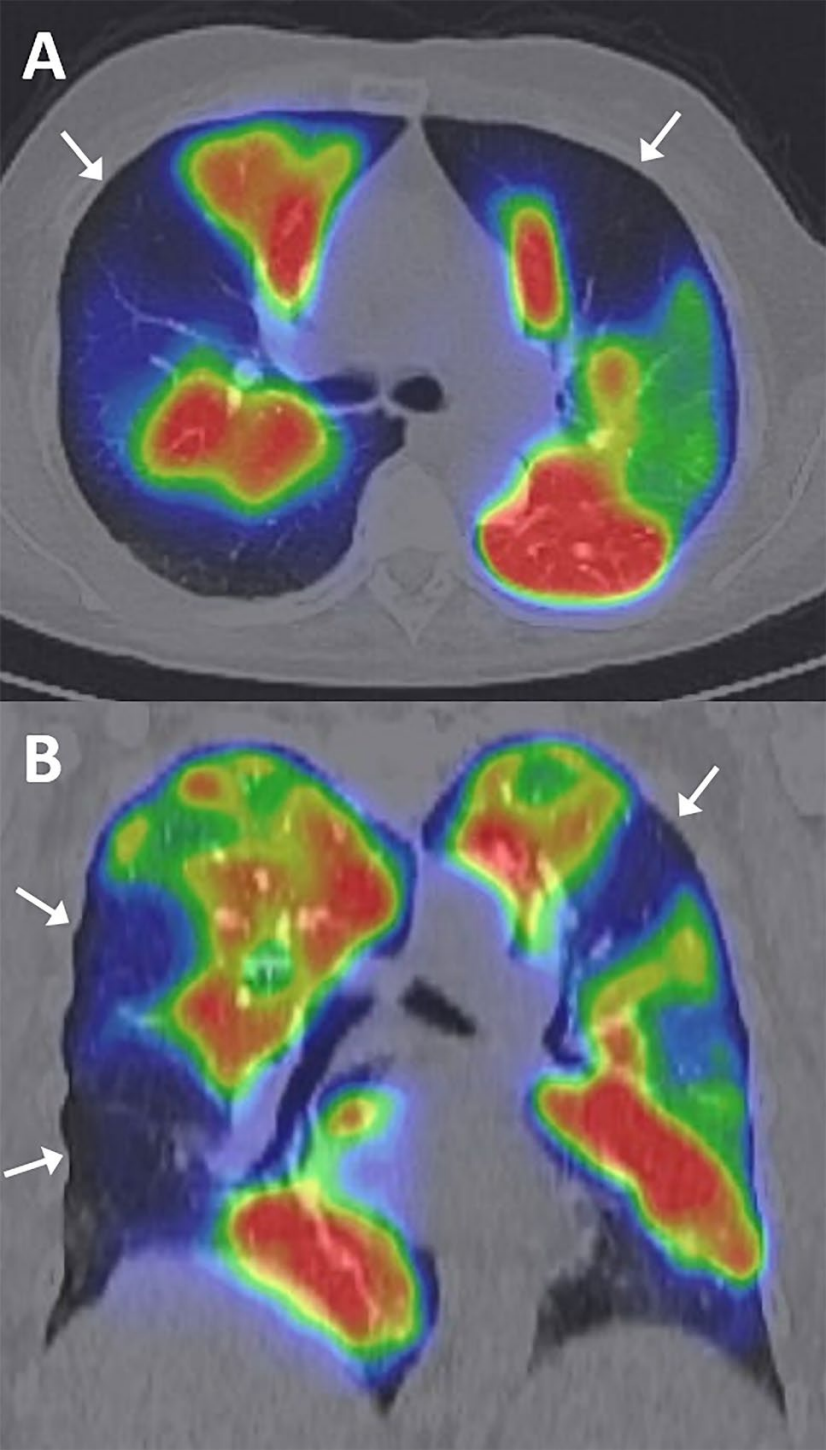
Representative perfusion SPECT/CT fusion images of a patient with chronic thromboembolic pulmonary hypertension (CTEPH). Axial (A) and coronal (B) views demonstrate multiple segmental perfusion defects, particularly in the peripheral regions of both lungs (white arrows). These findings are characteristic of CTEPH. Ventilation SPECT imaging was not available at our institution
